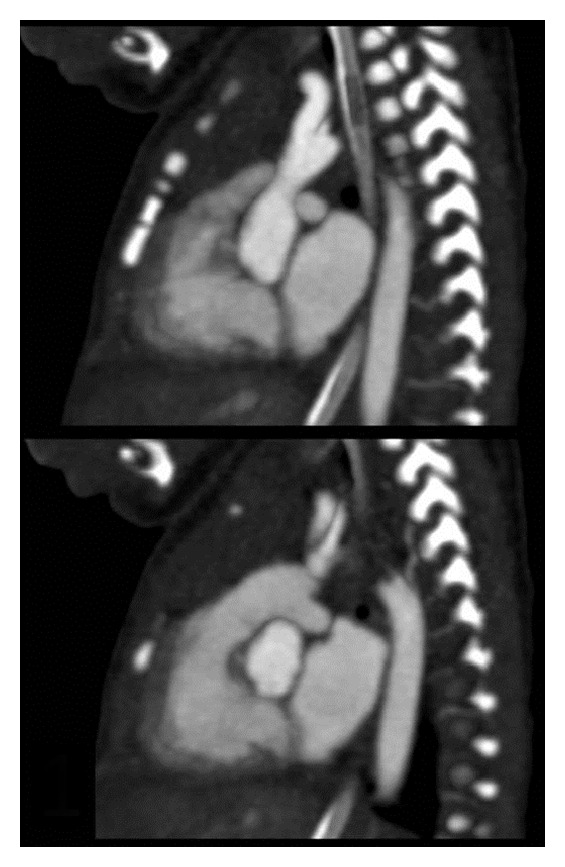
Computed tomography showing the aortic arch which appears to be interrupted with a radiodense lesion distal to the left subclavian artery.
